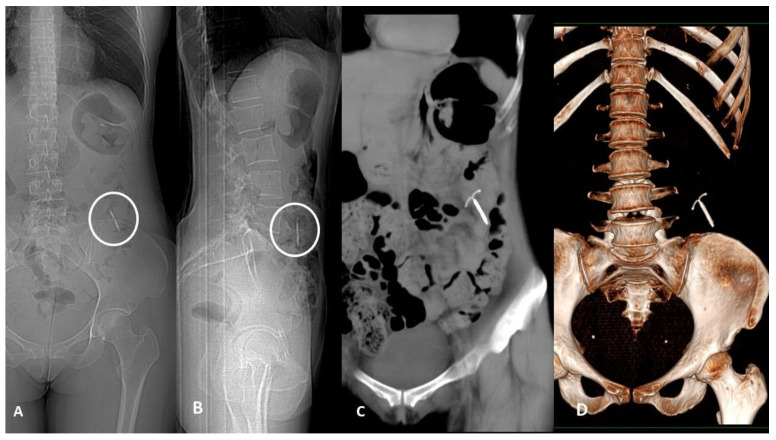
Device migration. Thirty-nine-year-old woman with acute left flank pain and history of copper Intra-uterine device (IUD) insertion 2 years earlier. Frontal (A) and lateral (B) CT scout of abdomen identify the IUD in the left lower quadrant. Non-enhanced CT scan coronal plane MIP-reformatted (C) shows device dislocation medially to descending colon in the pericolic fatty tissue; no displacement-related complications such as pneumoperitoneum or fluid collection were found. CT 3D-reconstruction (D).
